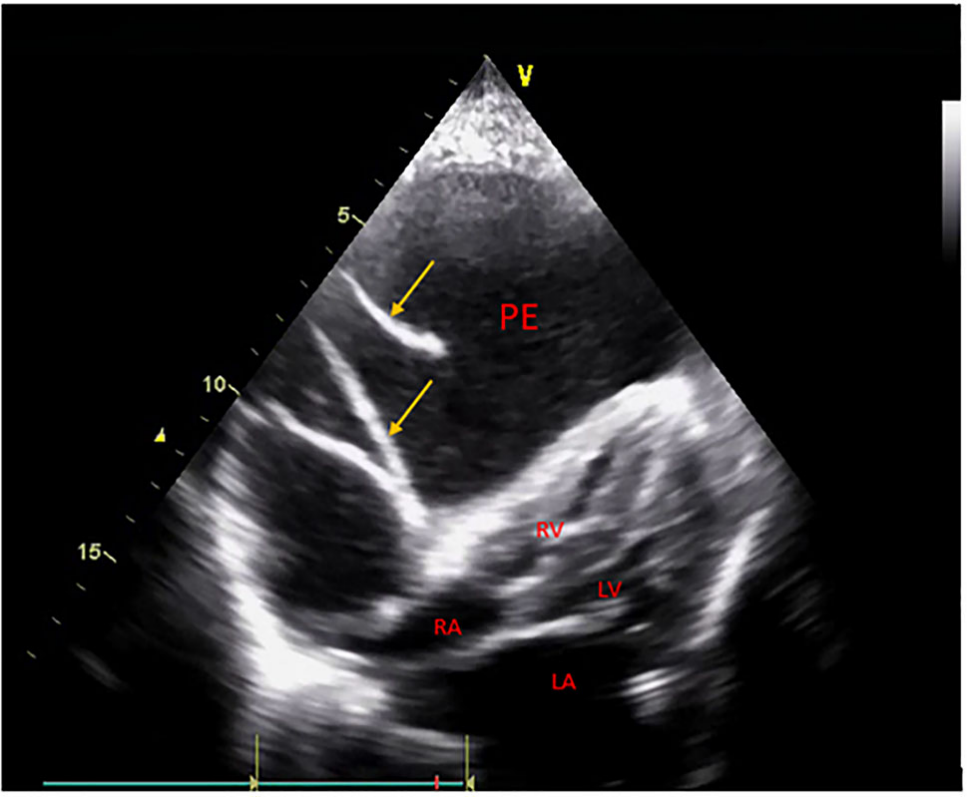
Echocardiogram showing a compressed heart (especially the right ventricle) due to a large pericardial effusion. Yellow arrows indicate fibre exudation bands. PE, pericardial effusion; RV, right ventricle; RA, right atrium; LV, left ventricle; LA, left atrium
